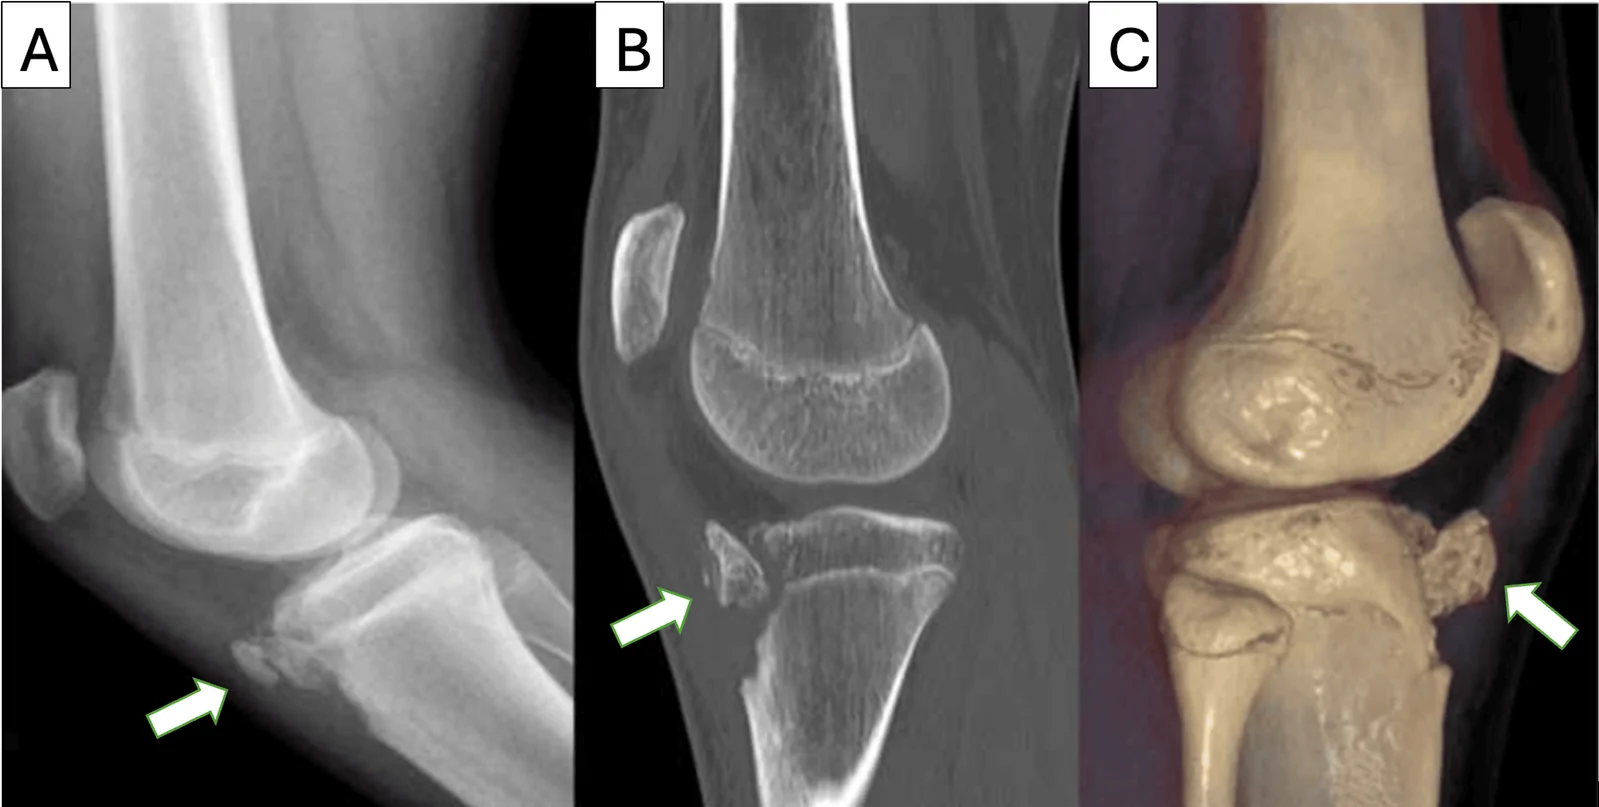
Preoperative imaging of case 2. (A) Lateral radiograph, (B) sagittal CT image, and (C) three-dimensional CT reconstruction demonstrating an Ogden type IB tibial tubercle fracture with complete rotational displacement of the fragment (arrows), suggestive of an associated patellar tendon avulsion.
